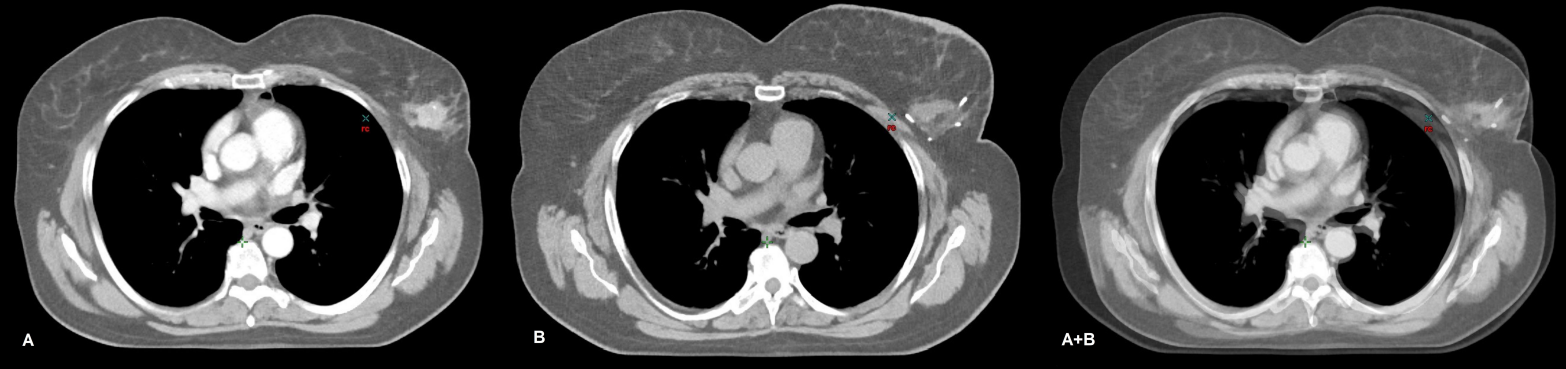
CT images of a participant in the CT scan cohort with cT2N0M0, G3 triple-negative breast cancer (TNBC) of the left breast. A contrast-enhanced focus is observed on the CT scan performed before systemic therapy (A). The planning CT of the same patient demonstrates the breast tumor bed and tracers inserted by the surgeon (B). Both images were taken in the same position. Although slight variations were observed in the respiratory phase, it does not affect the generation of fairly reliable fusion image by overlapping the two CT images (A+B). This approach may be facilitated while contouring the boost volume (increased irradiation dose).
